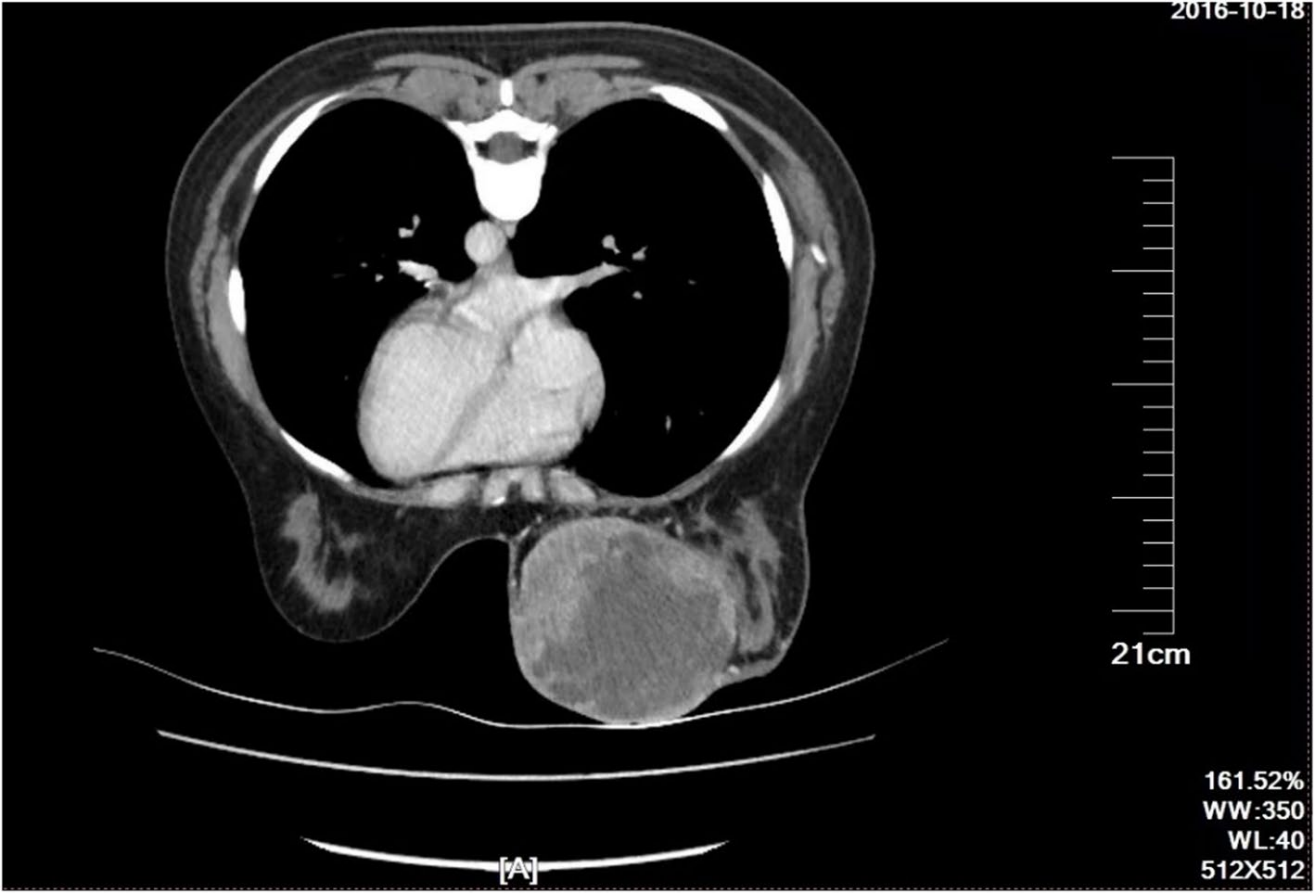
CT scan of the breast tumor. Breast CT revealed an irregular mass (12.0 × 11.3 × 11.7 cm) with local mixed echoes and marginal vessels formation. Left axillary lymph nodes are enlarged to 2.5 × 1.2 × 0.8 cm
